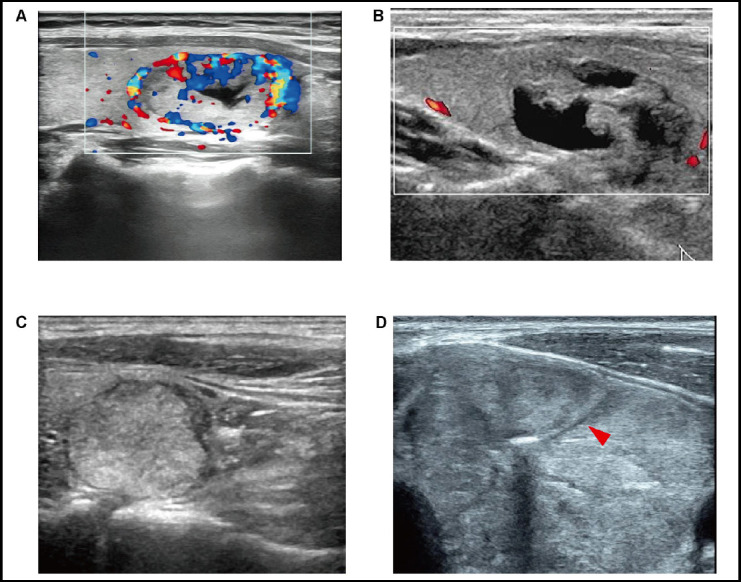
FTN and NG Ultrasound Images: A. Solitary thyroid adenoma with rich blood supply. B. Nodular goiter without peripheral halo, with relatively scarce blood supply. C. Thyroid follicular carcinoma with unevenly thickened peripheral halo. D. Thyroid follicular carcinoma showing the “separated nodule sign” (Red arrow).
